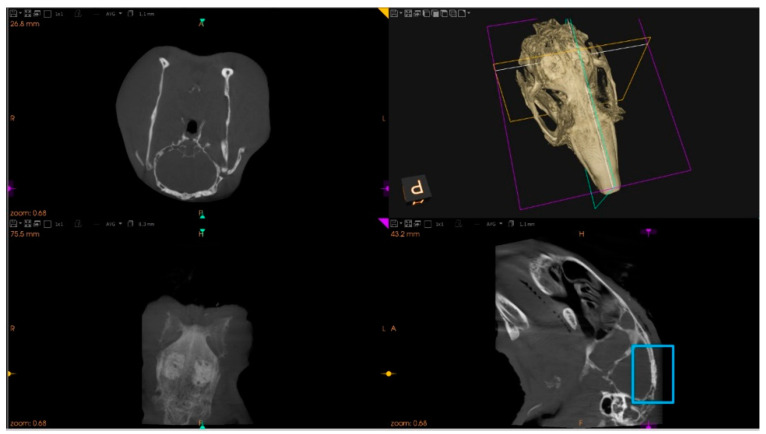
Cone beam computer tomography scan of the skull, done immediately after the surgical procedure.
